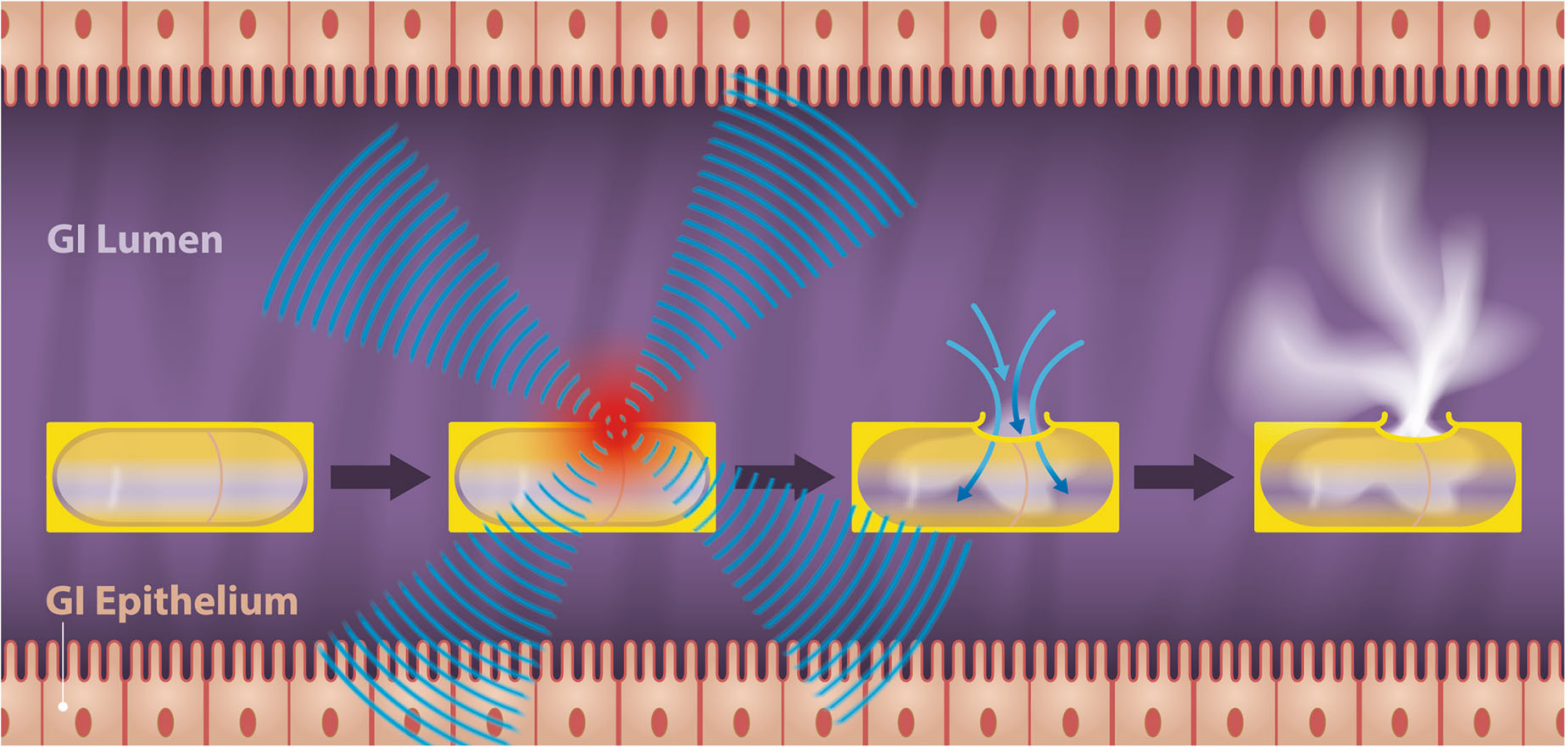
Graphic description of the HIFU-triggered drug release of a wax-coated capsule. Conceptually, once the wax-coated capsule is in the body (*e.g.* in the GI tract), it can be tracked due to its hypointensity on T2-weighted MRI (Fig. 5a). While the wax coating confers resistance to a premature release of the capsule content, its melting point can be designed to be low enough (in this study 43 °C) for a mild non-invasive external heat trigger (*e.g.* by HIFU) to melt a cavity into the coating. Water influx from the gut lumen [59] into the capsule core dissolves the lyophilised GBCA, leading to a well-visible hyperintense signal (white cloud) on T1-weighted MRI. GBCA: Gadolinium-based contrast agent; GI: Gastrointestinal; HIFU: High-intensity focused ultrasound; MRI: Magnetic resonance imaging
